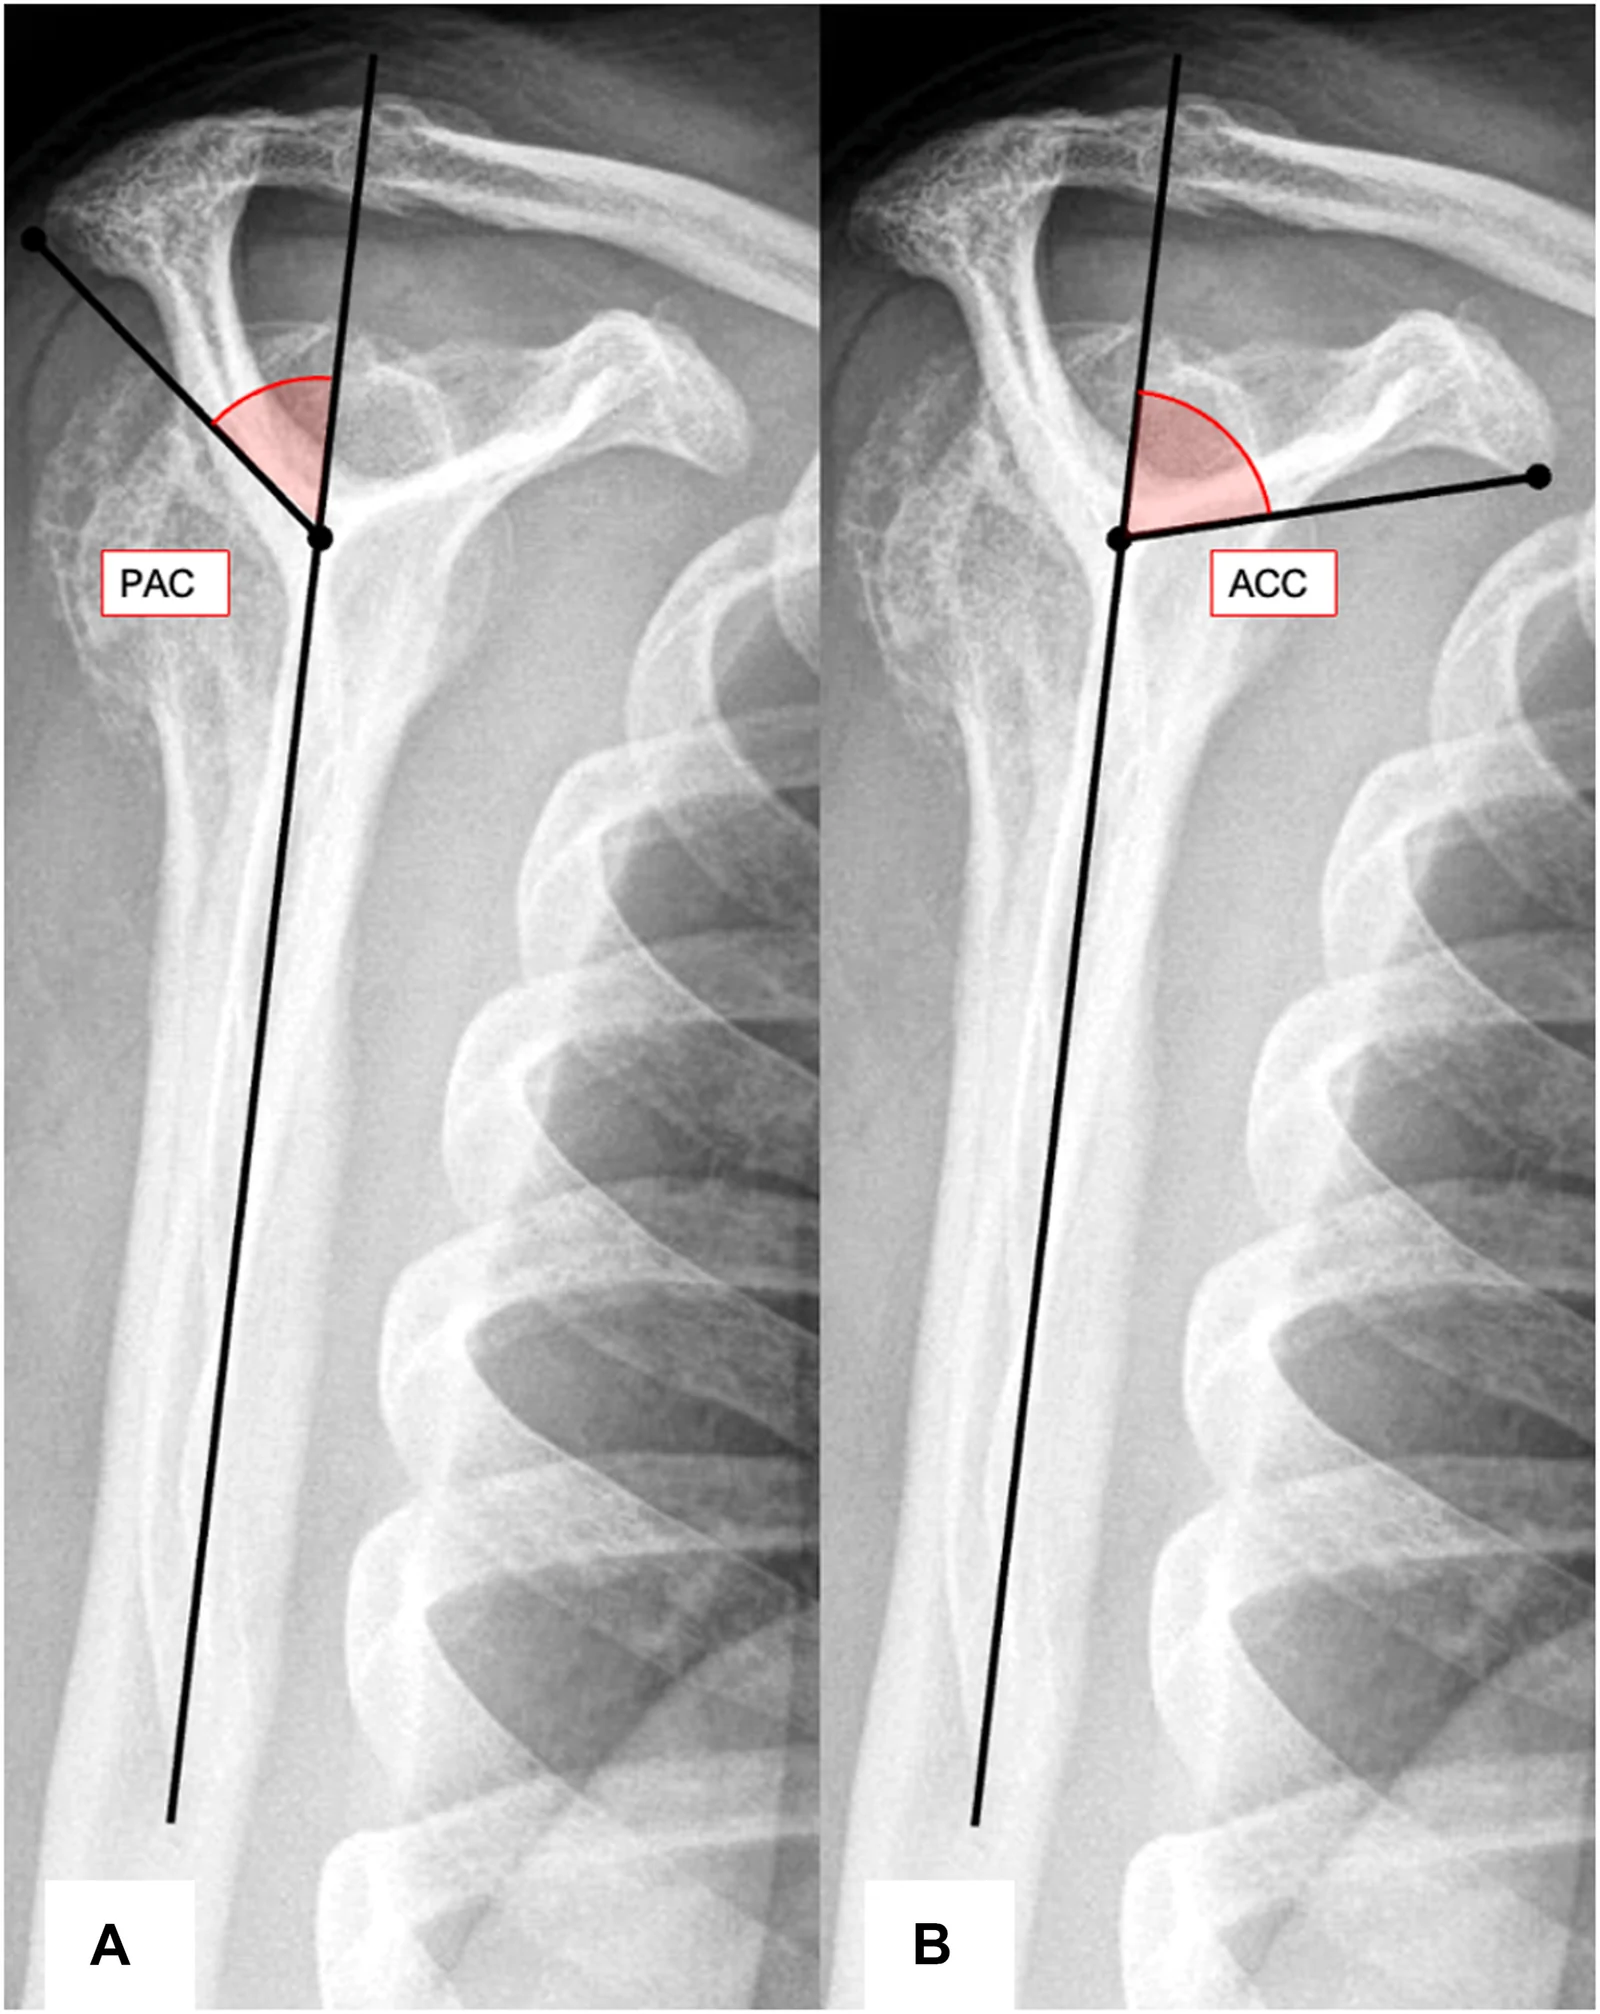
Measurement of PAC and ACC on standardized Neer lateral Y-view radiographs. (A) Posterior acromial coverage (PAC) was defined as the angle between the vertical scapular reference axis and a line drawn from the glenoid center to the most posterior-inferior cortical intersection point of the acromion. (B) Anterior coracoid coverage (ACC) was defined as the angle between the same vertical scapular reference axis and a line drawn from the glenoid center to the most inferior cortical point of the coracoid process. The glenoid center was defined as the best-fit intersection of the sclerotic cortical condensations of the scapular spine, coracoid, and scapular body.
